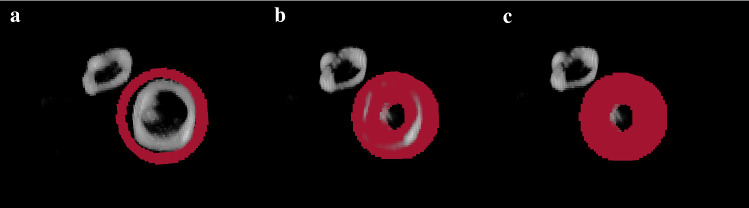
3D-visualization window to verify the adequation of the defined mask to the prosthetic ring obtained by means of the CT-based MIP subvolume. The user is expected to manually click and drag the volume to check for un-segmented valve regions. Moreover, the customization of the mask outer and inner radiuses (shown in a and b) and depth (shown in c) allows further refining the segmentation of the prosthetic ring
